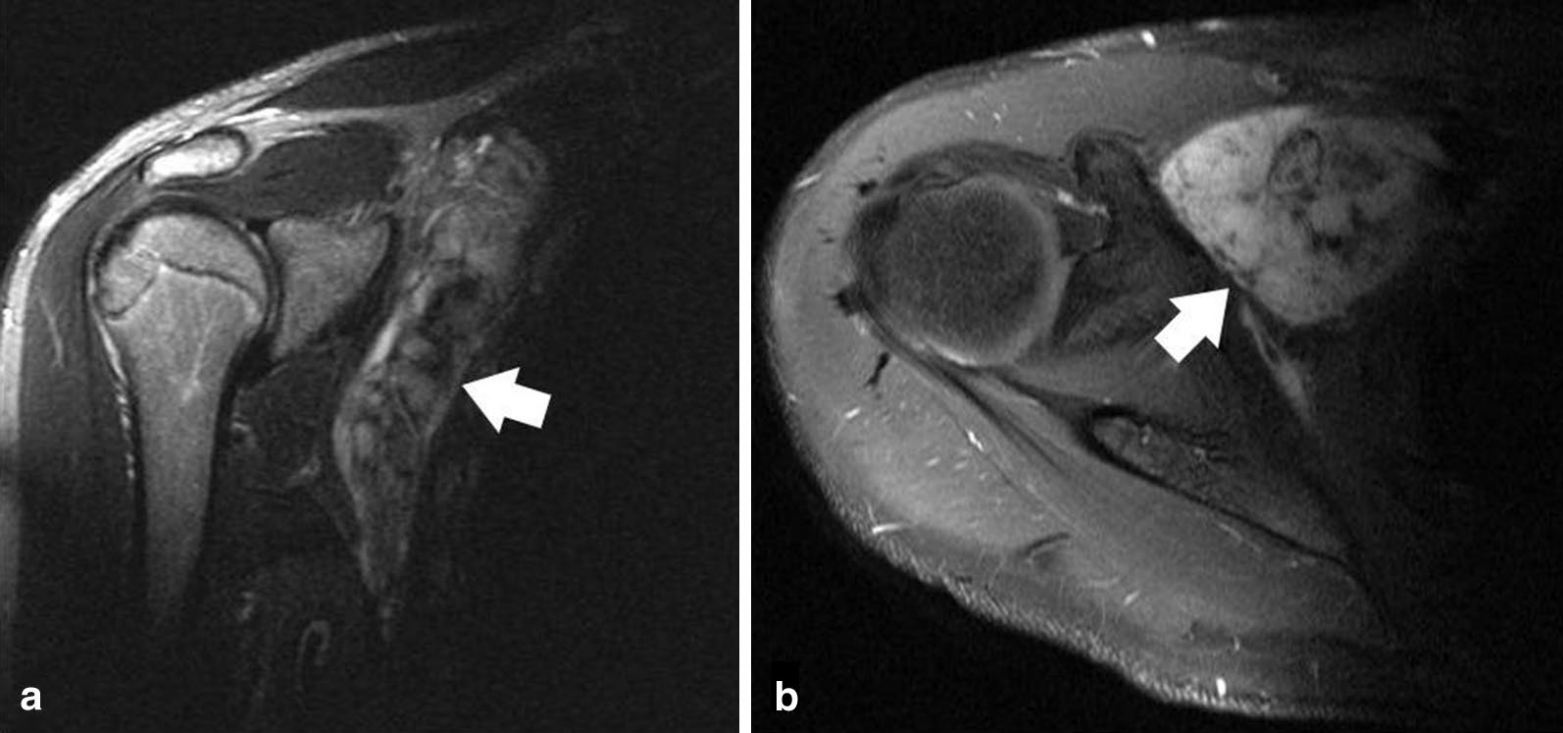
MRI of the right shoulder showing the tumor extent at the time of initial diagnosis in proton density (PD)-weighted (a) and T1-weighted contrast-enhanced (b) sequences. An inhomogeneously enhancing, partially fibrotic tumor (*white arrows*) originating from the right thoracic wall is clearly visible consistent with a desmoid-type fibromatosis
